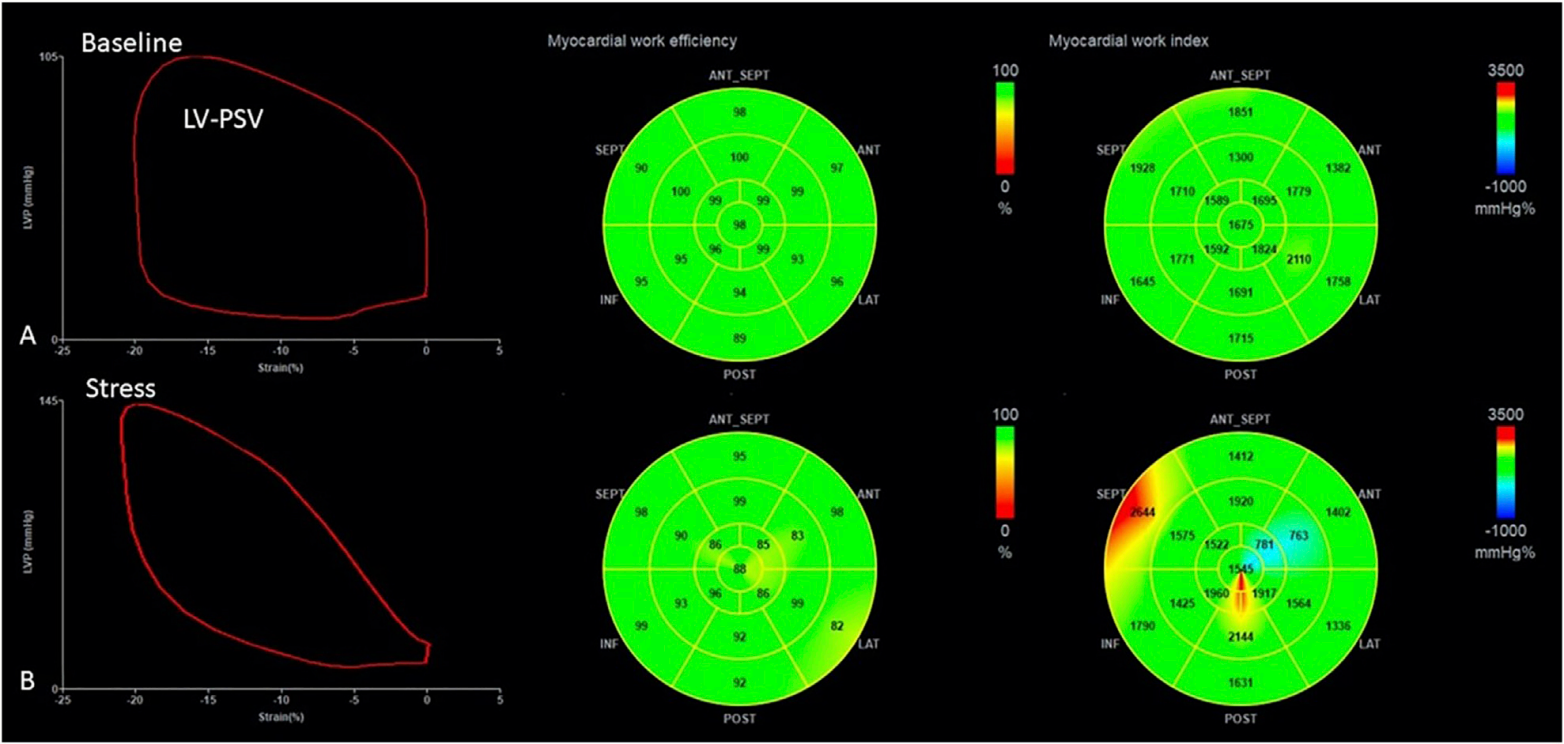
Measurement of myocardial work parameters by 2D echocardiography both at baseline (A) and during stress (B). The left panel demonstrates the generated LV pressure–strain loop; The middle and the right panels are bull’s eye plot of GWE and GWI, respectively. GWE, global work efficiency; GWI, global work index.
